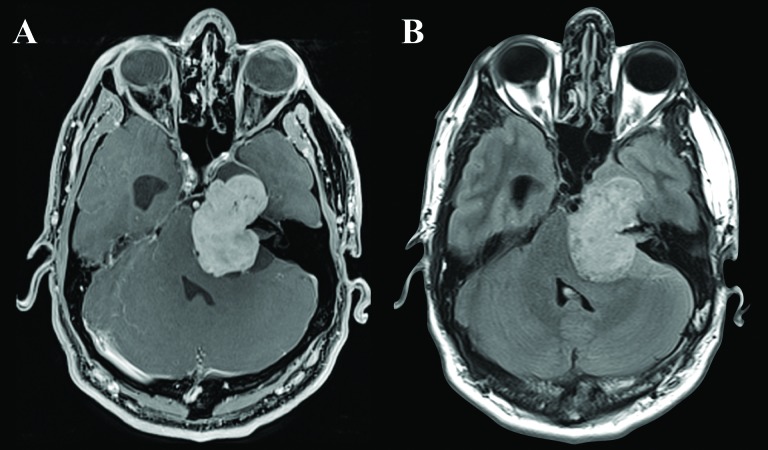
Preoperative MRI A. Axial T1-weighted magnetic resonance imaging (MRI) with gadolinium showing a homogenously enhancing extra-axial lesion, with significant mass effect on the brain stem, fourth ventricle, and left temporal lobe, with an enlarged right ventricular temporal horn. B. Axial fluid-attenuated inversion recovery (FLAIR) weighted MRI showing lack of edema in brainstem, cerebellum and left temporal lobe.
